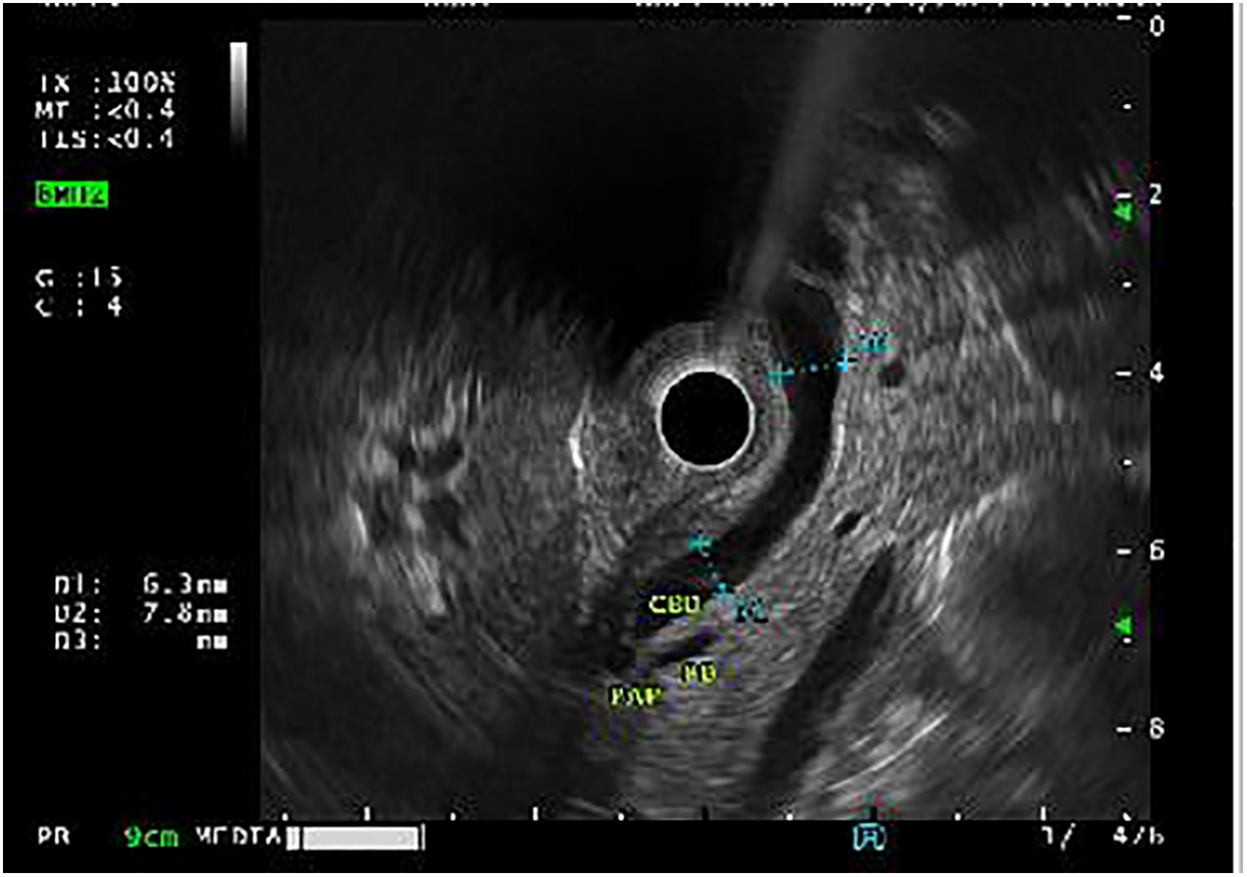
Endoscopic ultrasound (EUS) demonstrating absence of choledocholithiasis, averting endoscopic retrograde cholangiopancreatography (ERCP).
